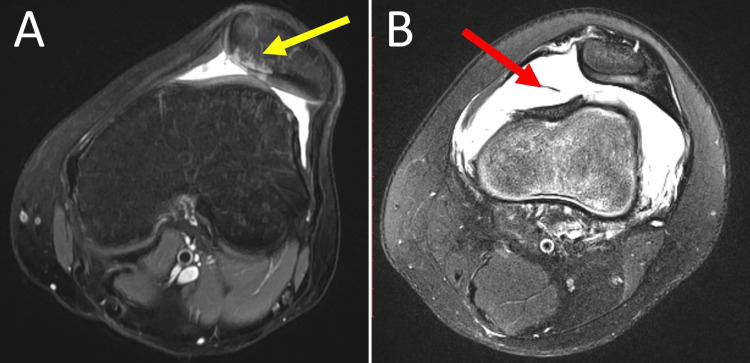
MRI of the knee in acute lateral PD (A) Arrow indicates bone bruising and chondral and osteochondral damage of the opposing articular surfaces. (B) Arrow indicates acute knee hematoma. Figure created by Panagiotis Samelis.
